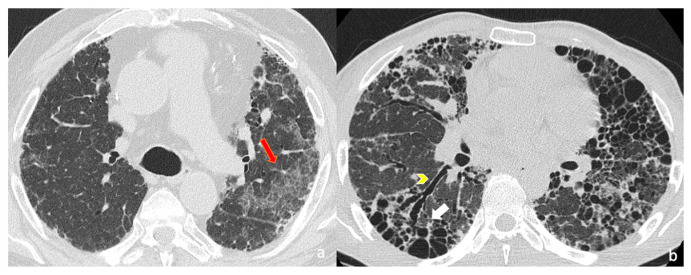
High-resolution computed tomography (HRCT) of the chest in axial (a,b) views. (a) Axial HRCT shows ground-glass opacities (subtle hazy increased attenuation) in left upper lobe, superimposed on fine reticulations (red arrow), suggesting fibrotic interstitial lung disease. (b) Advanced fibrotic changes are evident with traction bronchiectasis (yellow arrowhead) and extensive honeycombing (white arrow) in the posterior basal segments of the lower lobes, characterized by clustered cystic airspaces of similar diameter.
